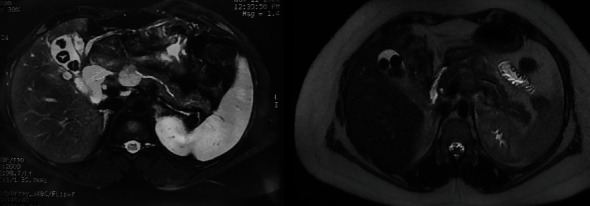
Preoperative MRI image of Frantz tumor. MRI, magnetic resonance imaging.
